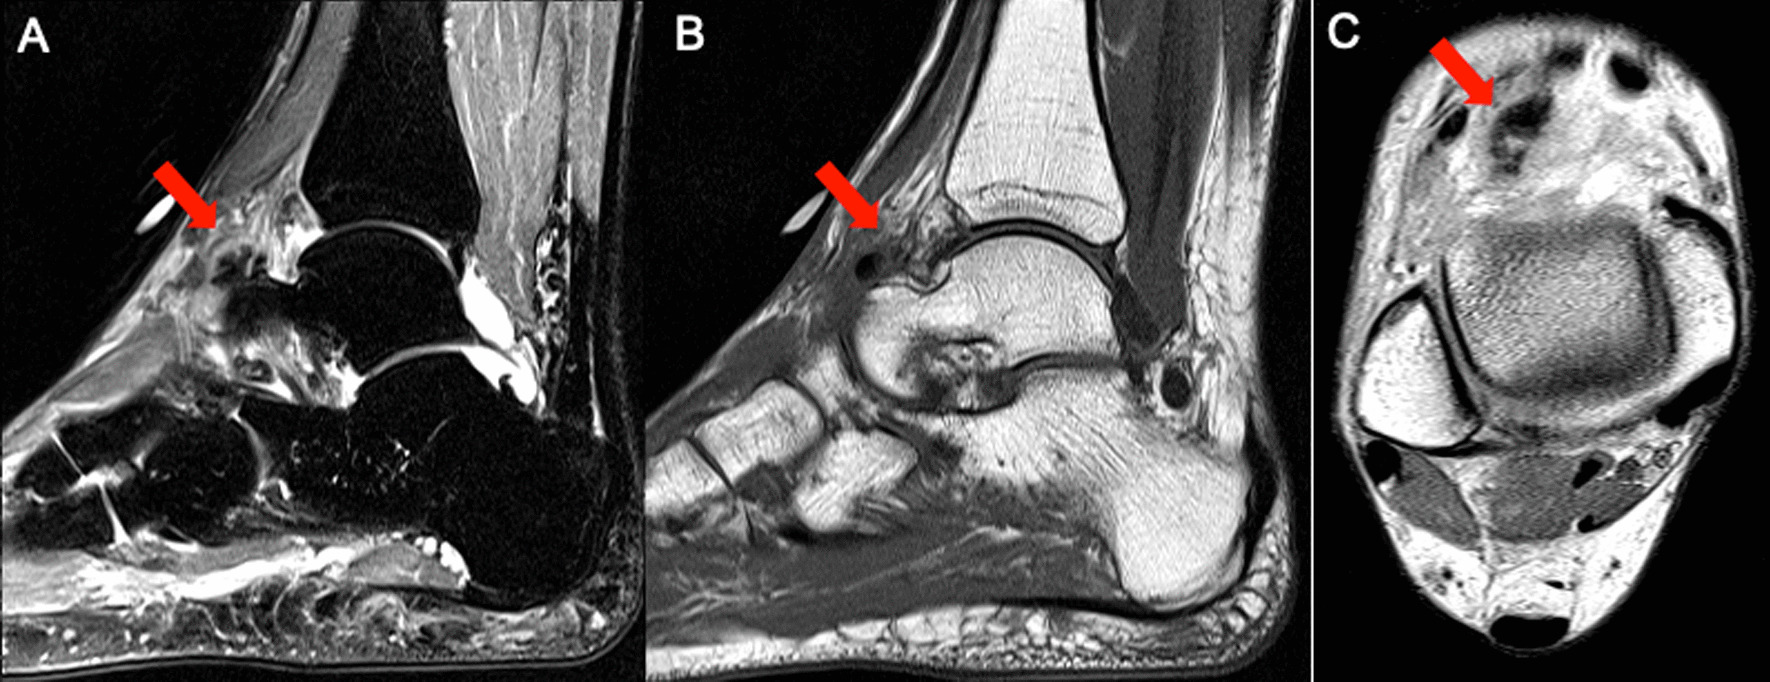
(A) Sagittal Short Tau Inversion Recovery, (B) Sagittal T1, and (C) Axial Proton Density Magnetic Resonance Images demonstrate a calcified soft tissue mass with surrounding inflammatory changes along the dorsal aspect of the talus, involving the inferior extensor retinaculum (frondiform ligament) as it passes along the extensor digitorum tendons, reflecting calcific Gruberi bursitis (arrows)
